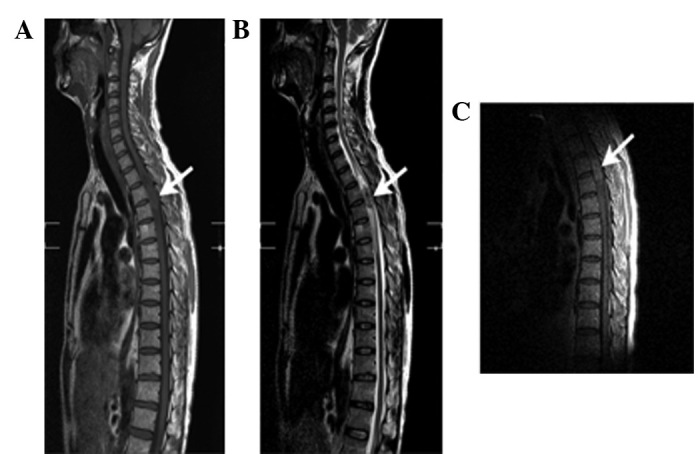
MRI images prior to surgery. (A) Abnormal ovoid long T1 and (B) long T2 soft tissue mass was observed at the level of third and fourth thoracic vertebra (white arrows). The size of the lesion was 1.3x1.1x2.4 cm with a clear margin and heterogenous intensity. (C) The lesion showed heterogenous contrast enhancement and compression of the adjacent subarachnoid space (white arrow).
